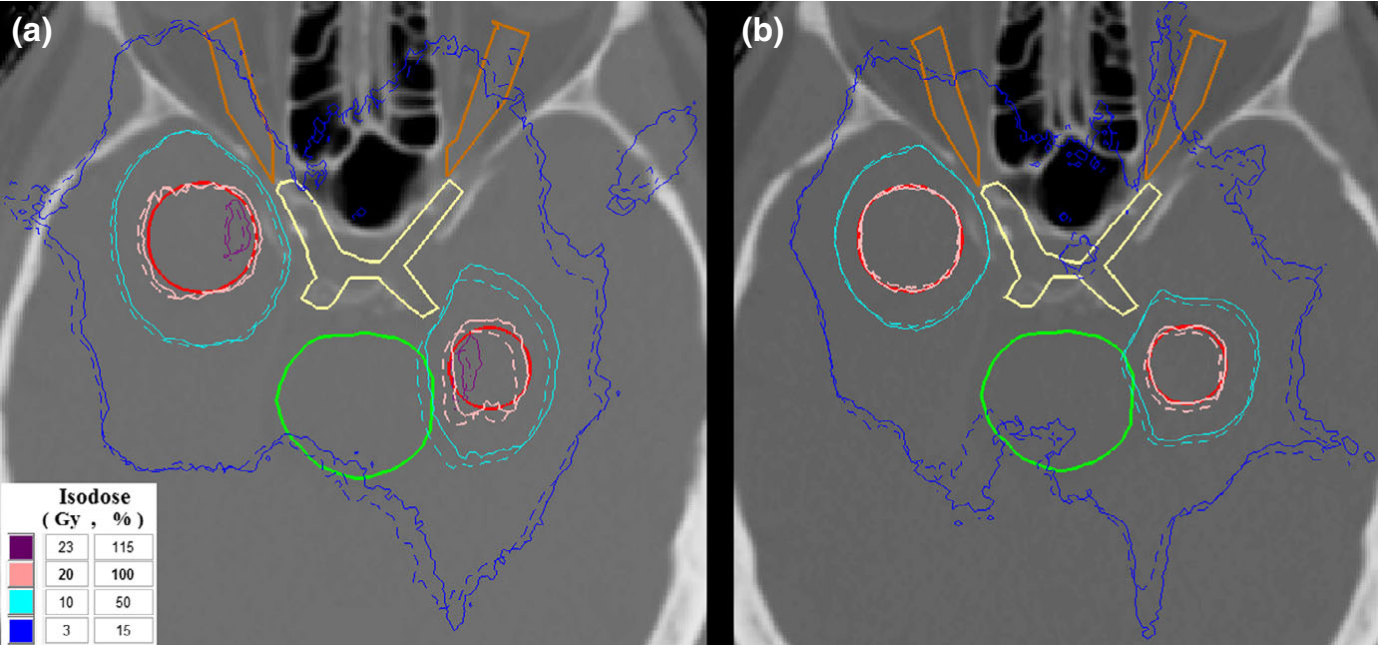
Axial CT slice of patient #5 with isodose lines superimposed for the (a) single‐ and (b) two‐isocenter techniques. Reference dose distributions are represented by solid lines, whereas dashed lines correspond to rotated dose distributions (2°, around all three axes). Contours legend: targets: red, brainstem: green, optic chiasm: yellow, optic nerves: brown.
